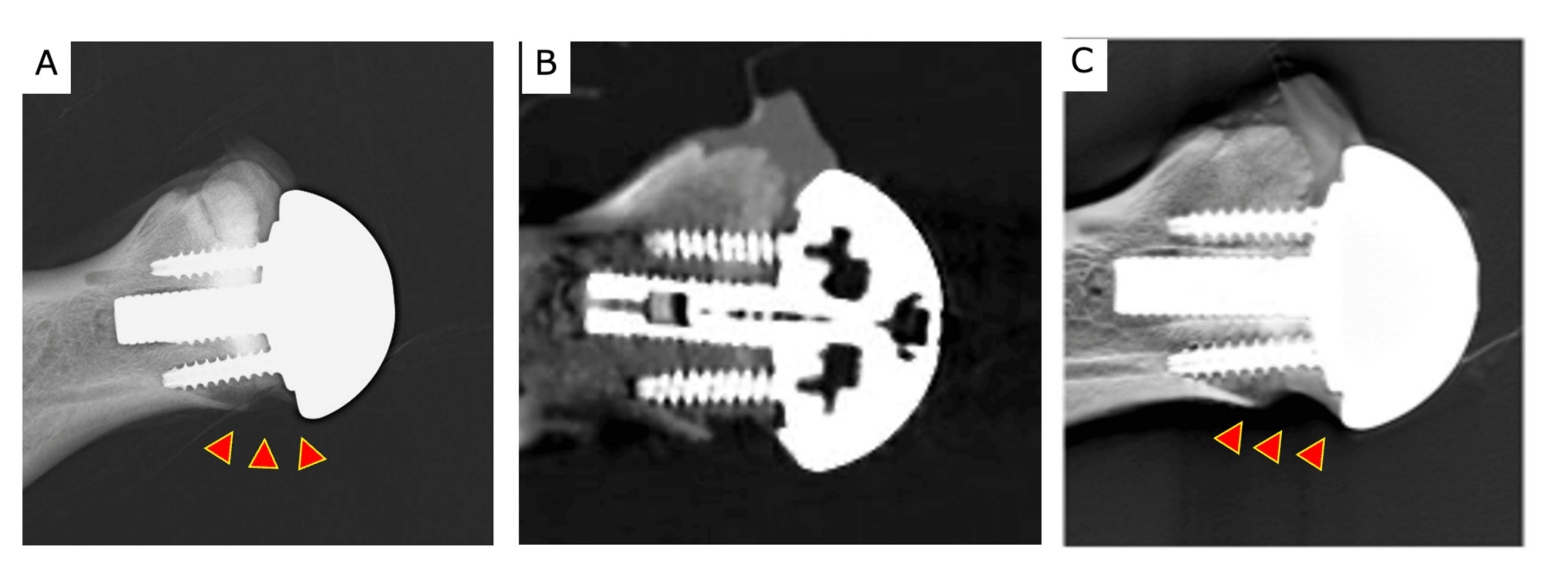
Inferior glenoid bone defect models a. radiographic evaluation, b. coronal CT image, and c. tomosynthesis. Arrowheads show areas of the inferior glenoid bone defect.
